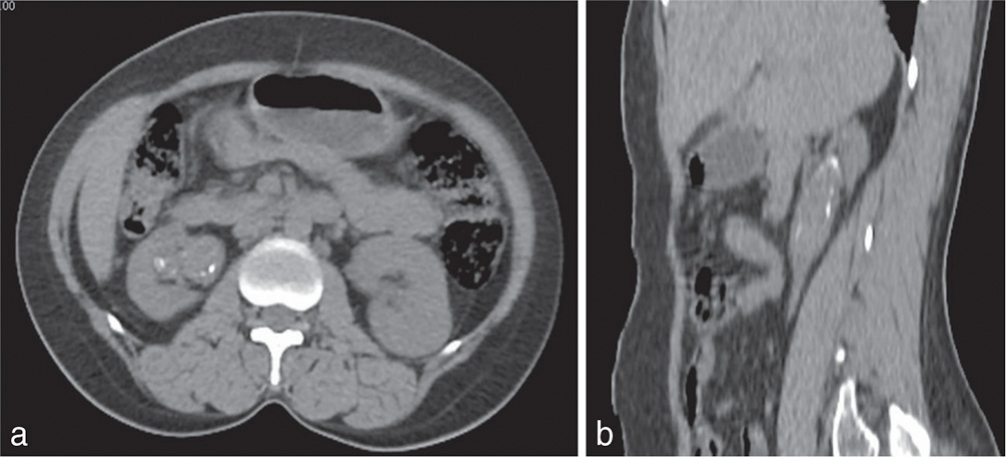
Plain CT scan axial (a) and sagittal (b) reconstruction showing an isodense mass lesion with a peripheral rim of calcification in the dilated renal pelvis.
